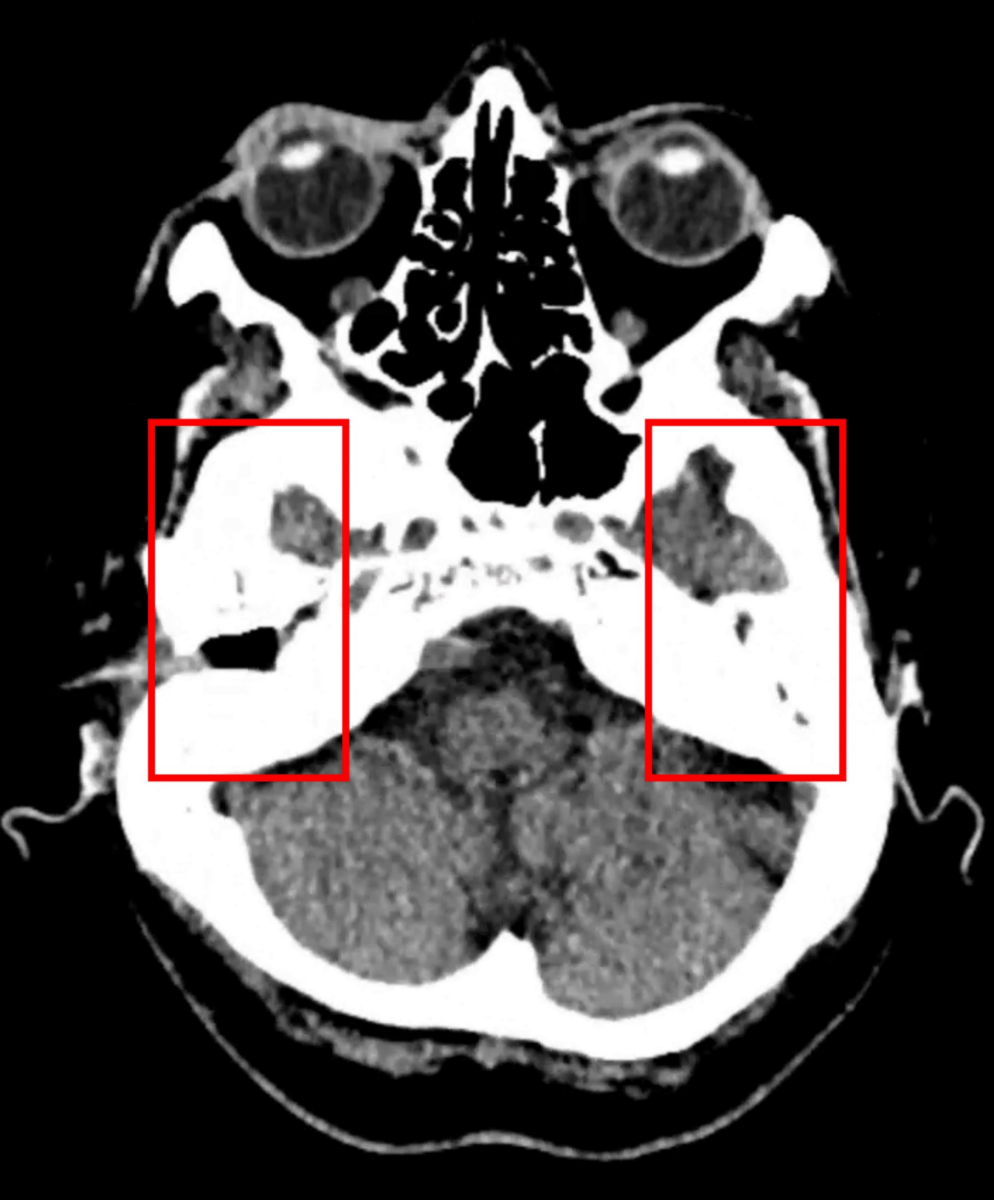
Axial CT showing middle ear cavity opacification Opacification of the middle ear cavities, more marked on the right, without evidence of coalescent mastoiditis or ossicular erosion.
